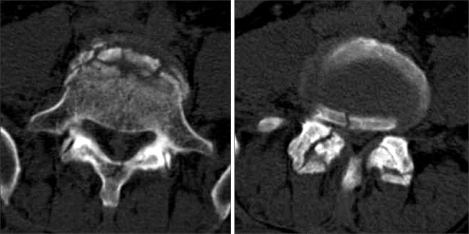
Computed tomography showed an unstable burst fracture of L5. Fracture of the L5 body with a greenstick lamina fracture (A) and bilateral inferior articular process fracture of L4 (B).
